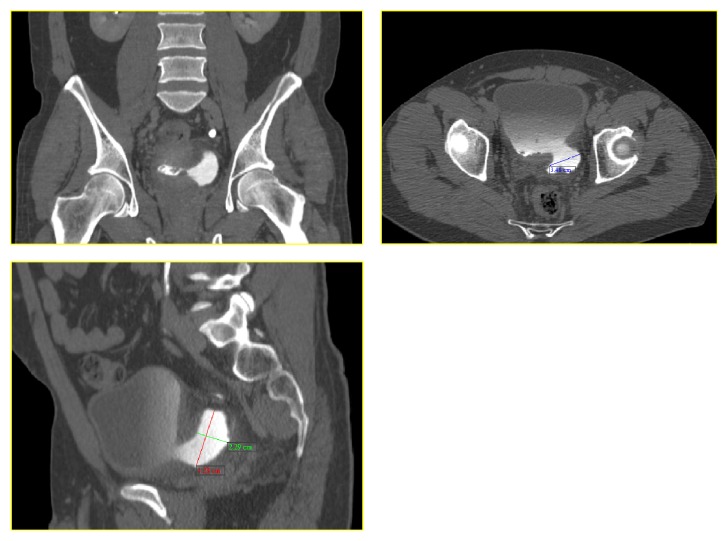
Coronal, sagittal, and axial cuts of bladder diverticulum on computed tomography.
